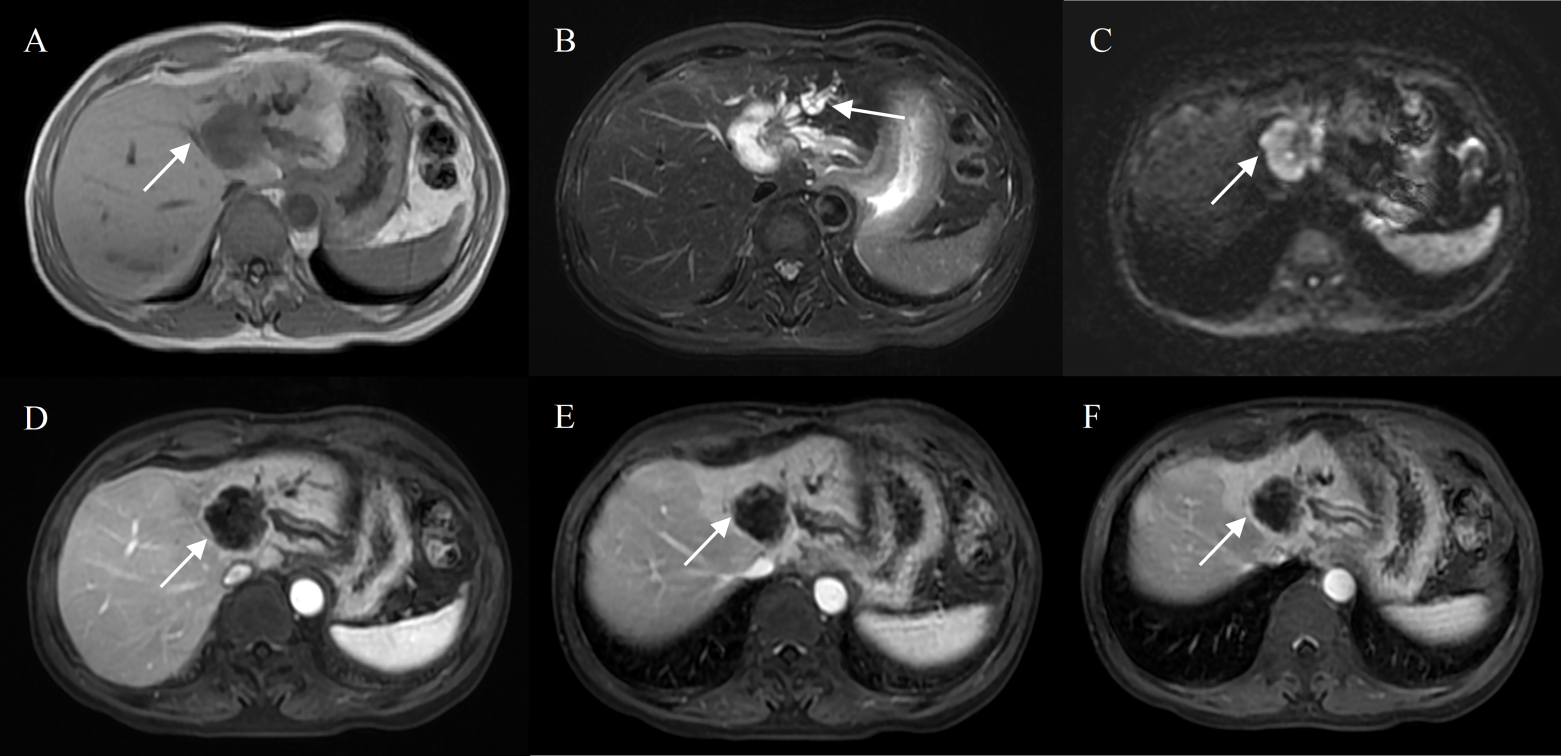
Intrahepatic mass-forming cholangiocarcinoma in a 70-year-old male without chronic viral hepatitis. Gd-DTPA-enhanced MRI detected a lobulated tumor (4.3 cm) with homogeneous SI on T1WI and T2WI (A, B), restricted diffusion (C). Peripheral rim-like enhancement in the arterial phase (D), sustained rim enhancement in the portal and delayed phase (E, F). And bile duct dilation peripheral to the tumor (B).
